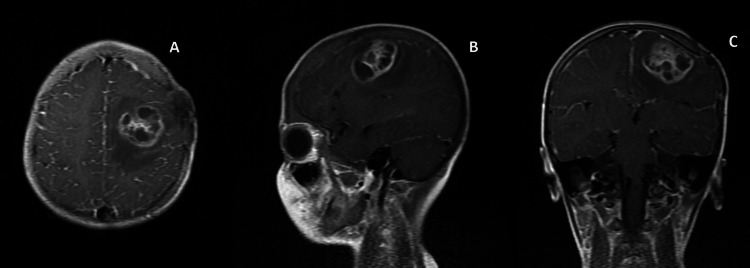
Axial (A), Saggital (B), Coronal (C) Contrast MRI brain showing a large, thick-walled, multi-septate peripheral enhancing lesion in the left high frontoparietal region measuring around 2.1 x 3.2 x 3.5 mm (CCxTRxAP) with associated perilesional oedema.
